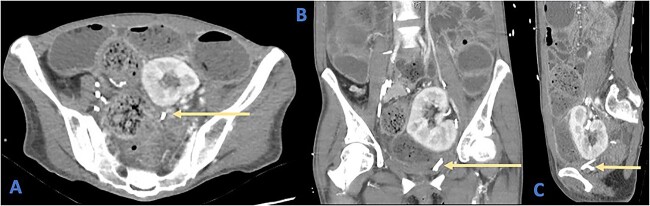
Axial (A), coronal (B), and sagittal (C) views of a CT demonstrating the position of the ureteric stent (arrows) as it crosses the rectosigmoid colon. Note the faecalization of proximal colon and dilated colonic loops.
